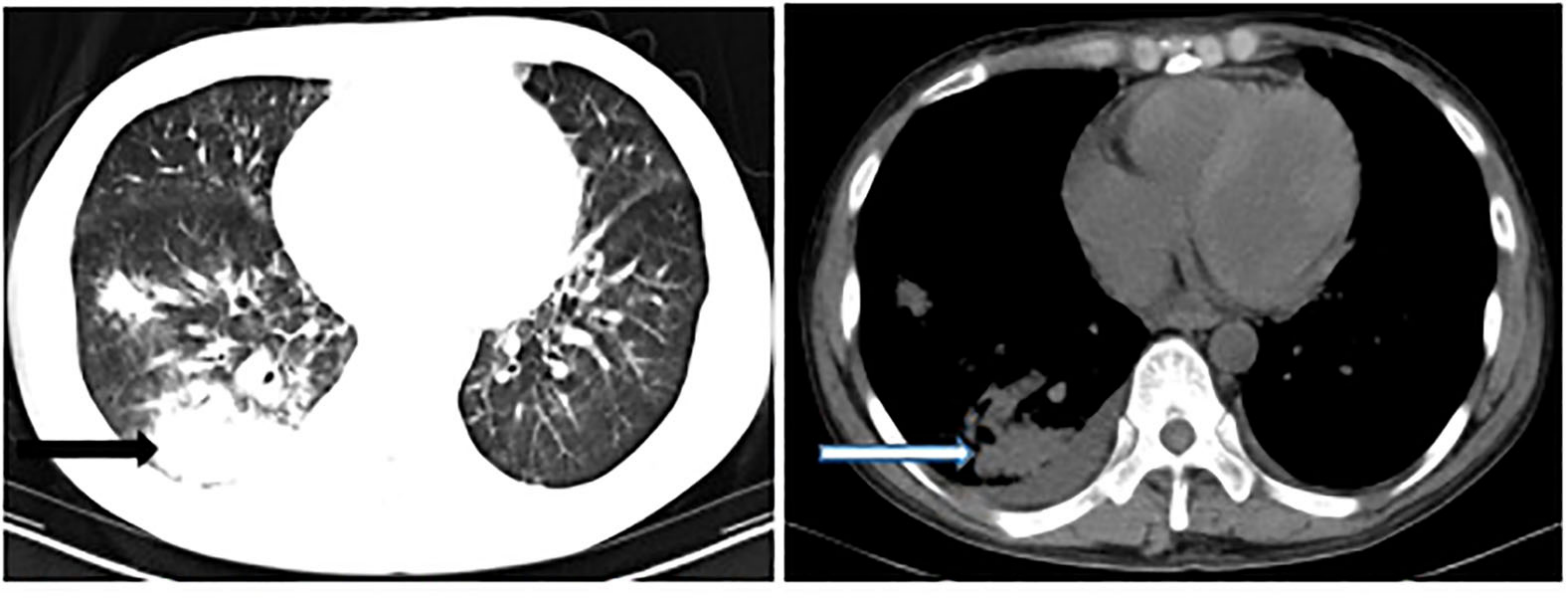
Computed tomography examination of the lungs showed multiple patchy shadows, especially in the right lung (arrow).
